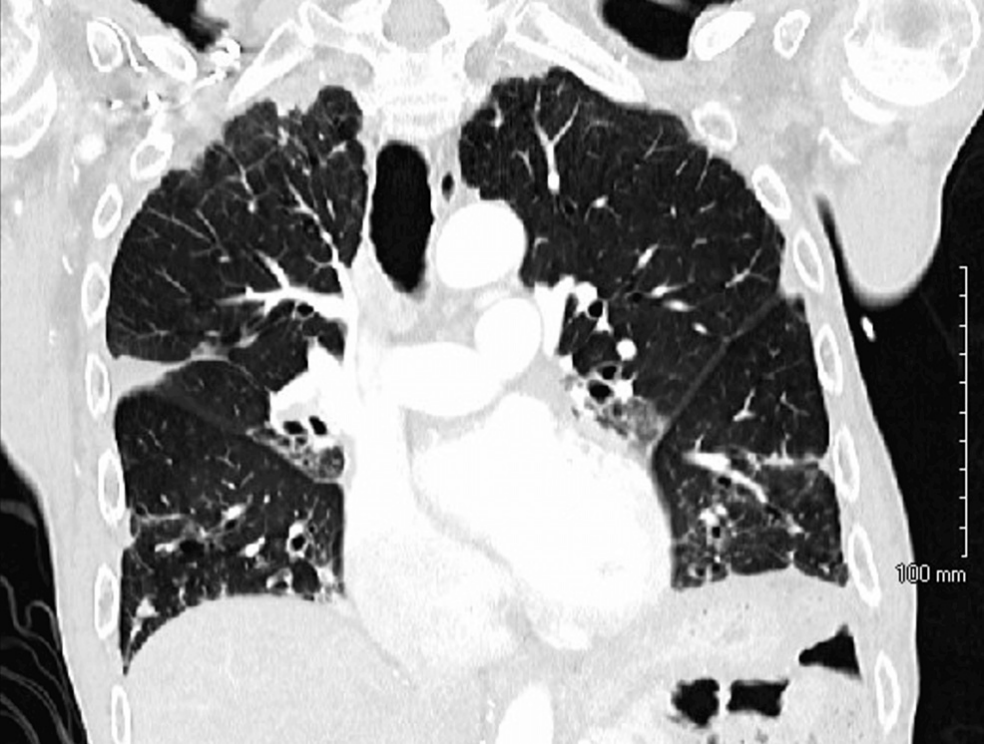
CT of the chest with contrast (coronal view) showing bronchiectasis in the lingula, right middle lobe, and lung bases.
